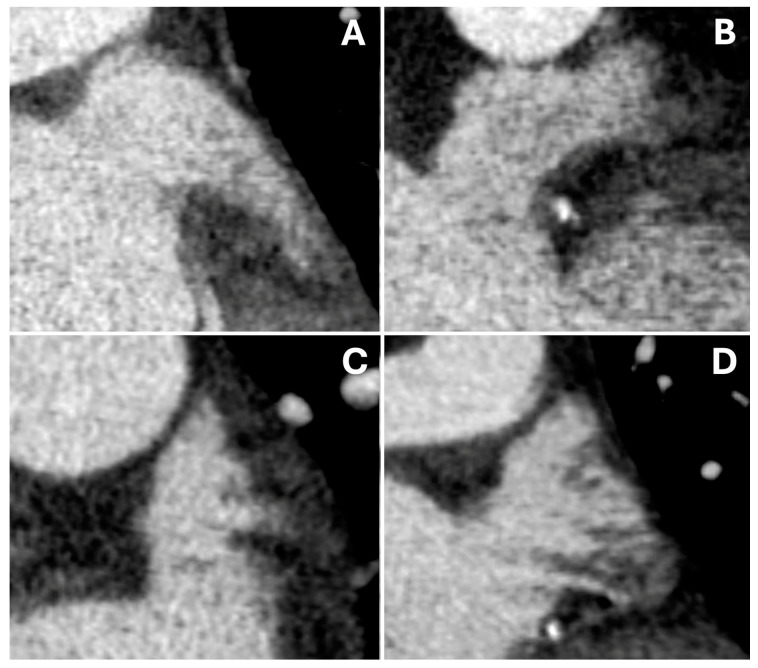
Representative examples of each left atrial appendage type on computed tomography angiography: (A) chicken-wing, (B) windsock, (C) cactus, (D) cauliflower.
